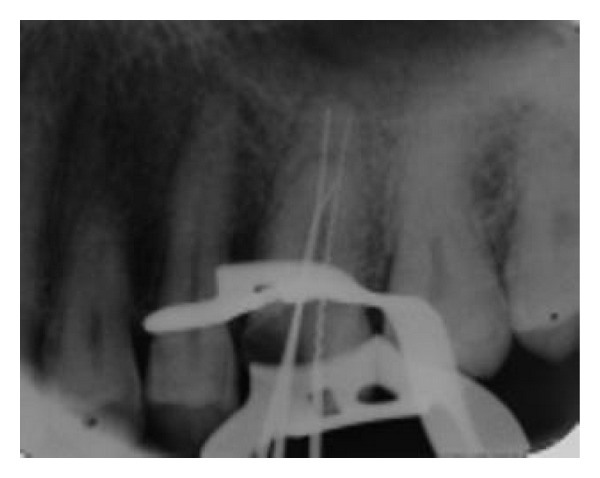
Length-determination radiograph.
